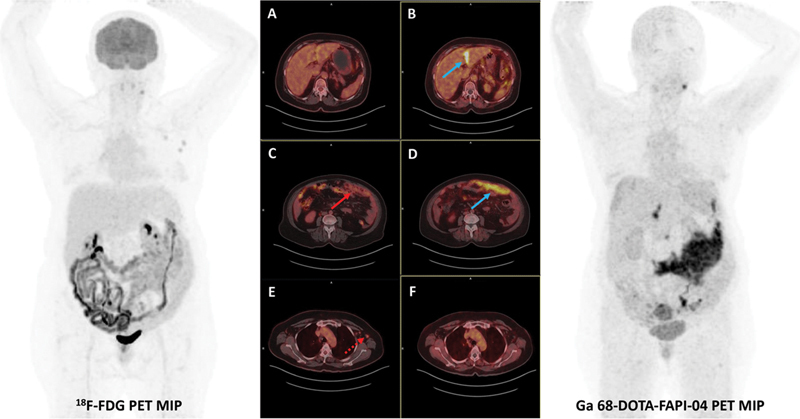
A 57-year-old woman showing low FDG uptake (SUVmax = 3.9) on the liver capsule surface but intense uptake with FAPI (SUVmax = 15.1), (
